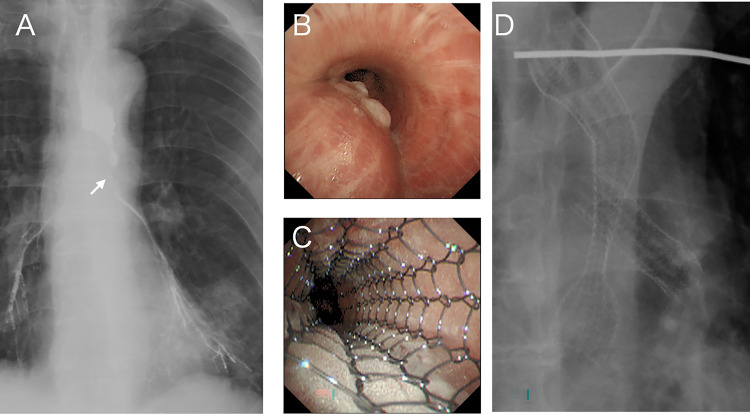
Radiological and endoscopic findings of case 3: Fistulography showing the esophago-bronchial fistula (arrow, A); bronchoscopic image showing tumor invasion in the left main bronchus (B) and the inserted bronchial stent (C); A chest X-ray showing the “double stenting, “namely, both bronchial and esophageal stenting (D).
